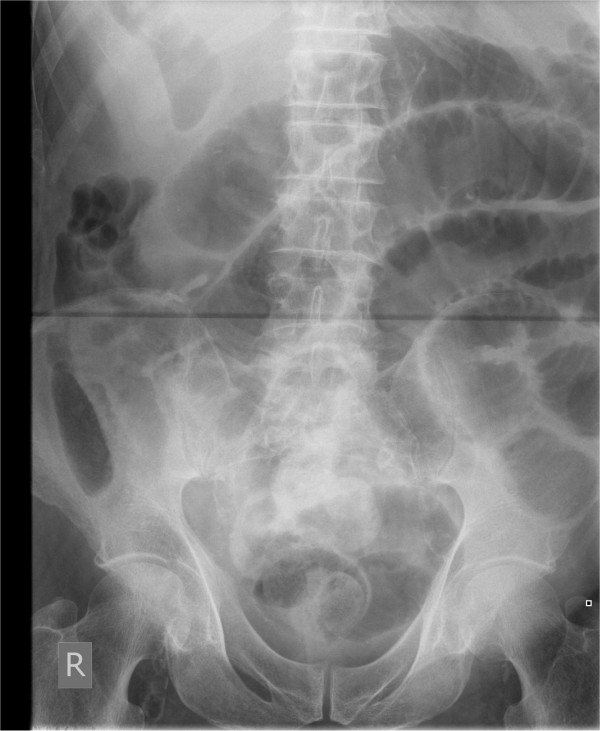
Abdominal X-ray of patient 1 showing dilated loops of small bowel.
